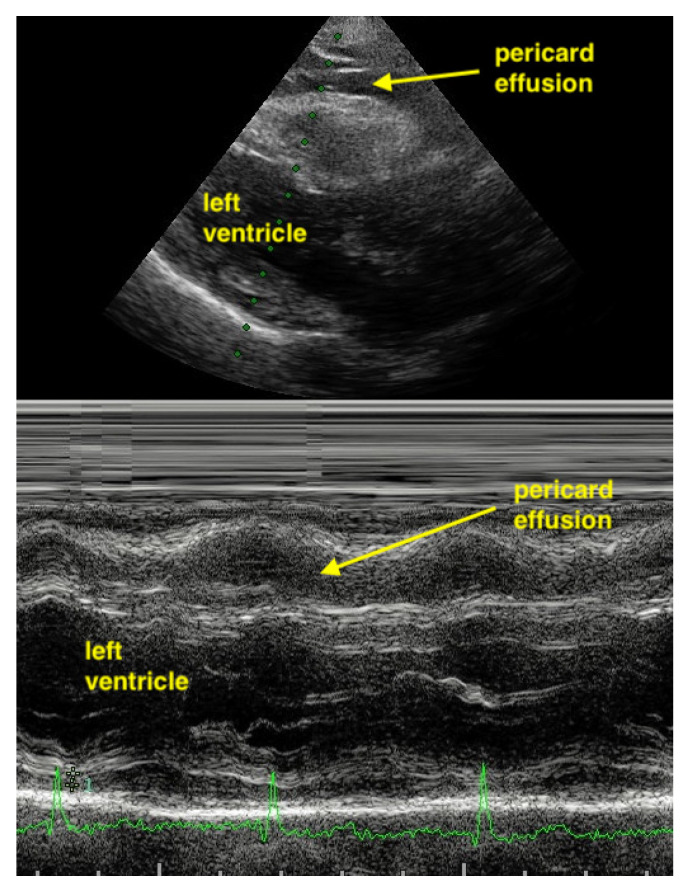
In the parasternal long-axis view, the hypoechoic band of the pericardial effusion varying in size during heart contraction (undulating).
